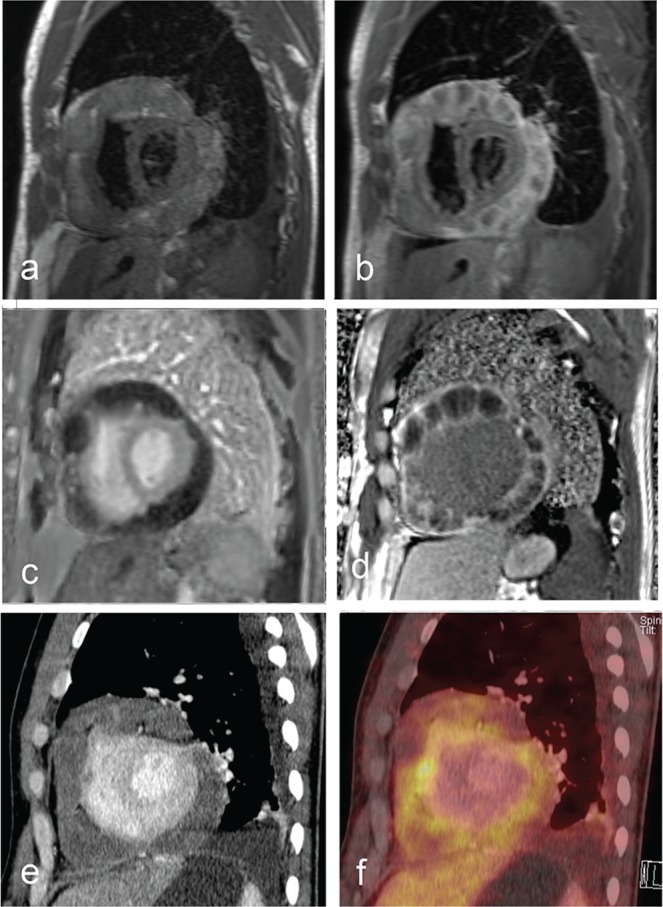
Comparison of different imaging techniques in cardiac short axis plane. (a) Non-contrast-enhanced T_1_-weighted (T_1_-w) “dark blood” TSE, (b) contrast-enhanced T_1_-w “dark blood” TSE, (c) early-enhancement inversion-recovery SSFP, (d) late-enhancement inversion-recovery SSFP, (e) contrast-enhanced CT, and (f) contrast-enhanced CT and PET fusion. “Sun ray appearance” can be recognized on part (b) and (d), other techniques have failed to display this diagnostic pattern. CT, computed tomography; SSFP, steady-state free precession; PET, positron emission tomography; TSE, turbo spin echo.
